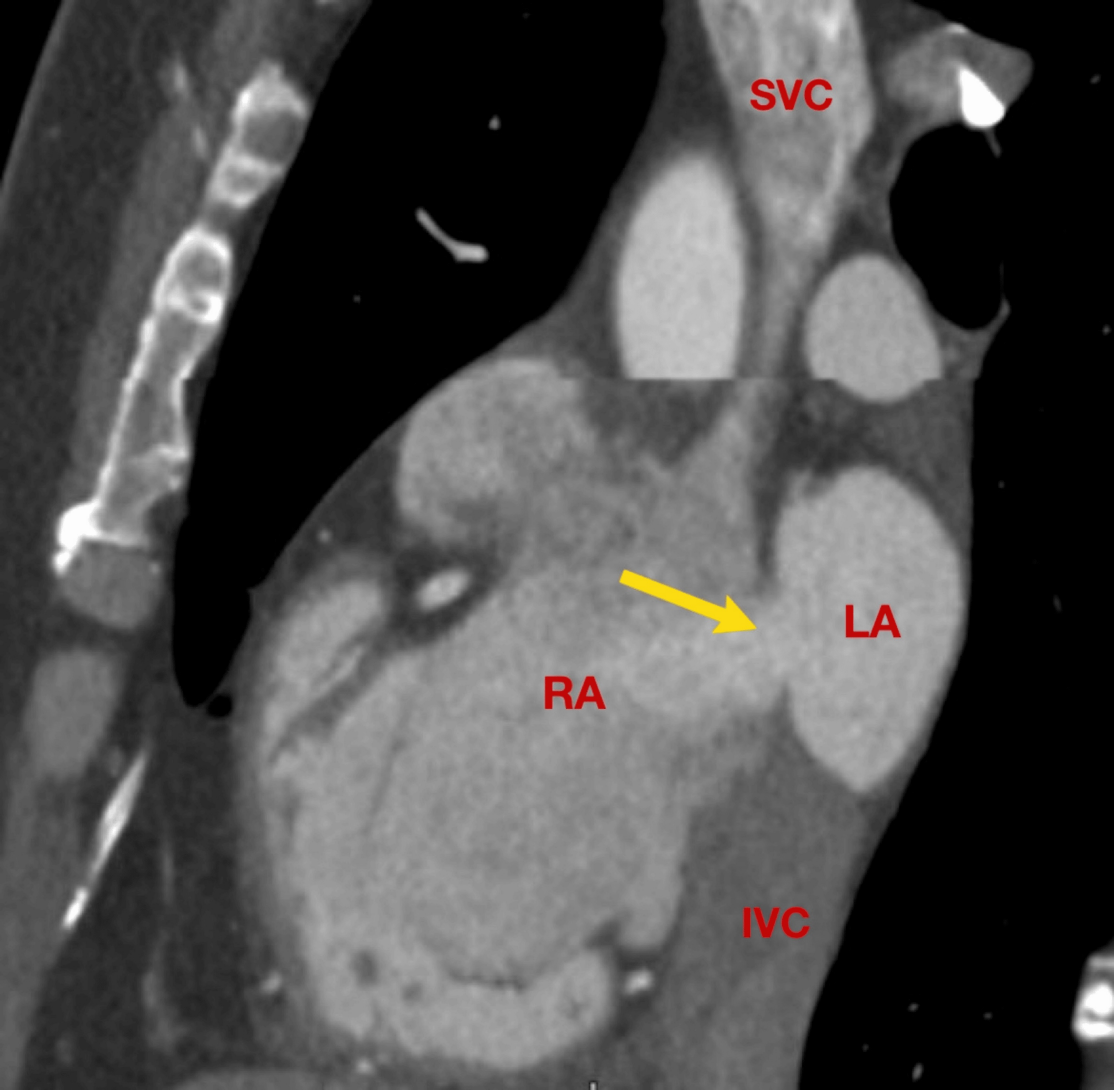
Multidetector CT (MDCT): multiplanar reformat view at the level of the atria, corresponding to the TEE bicaval view, demonstrating the atrial septal defect. TEE: transesophageal echocardiography; SVC: superior vena cava; IVC: inferior vena cava; RA: right atrium; LA: left atrium
